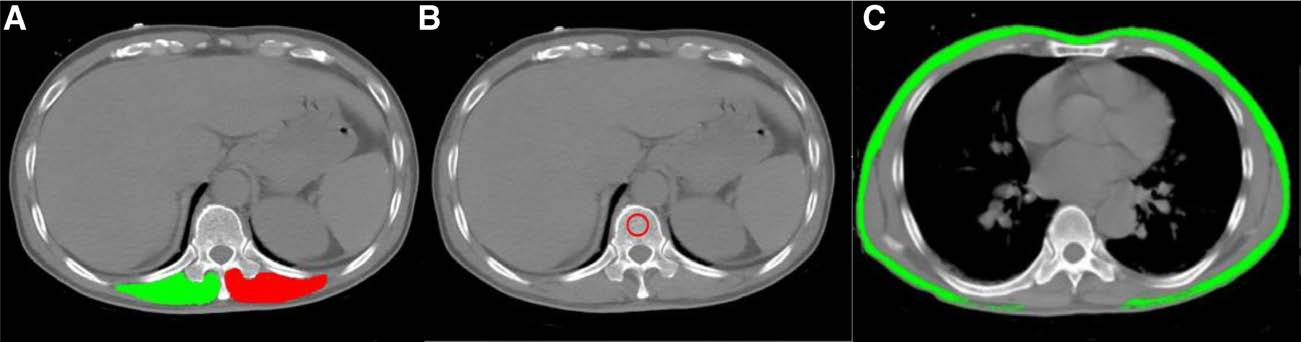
Sample CT scans used to determine muscle area in our cohort. (A) ESMA measured at the T12 level: green indicate right muscle and red, left muscle. (B) Bone density measured in the region of interest (ROI) indicated with red circle, at the T12 level. (C) SAT measured at the T7-8 level, highlighted in green. CT = computed tomography.
